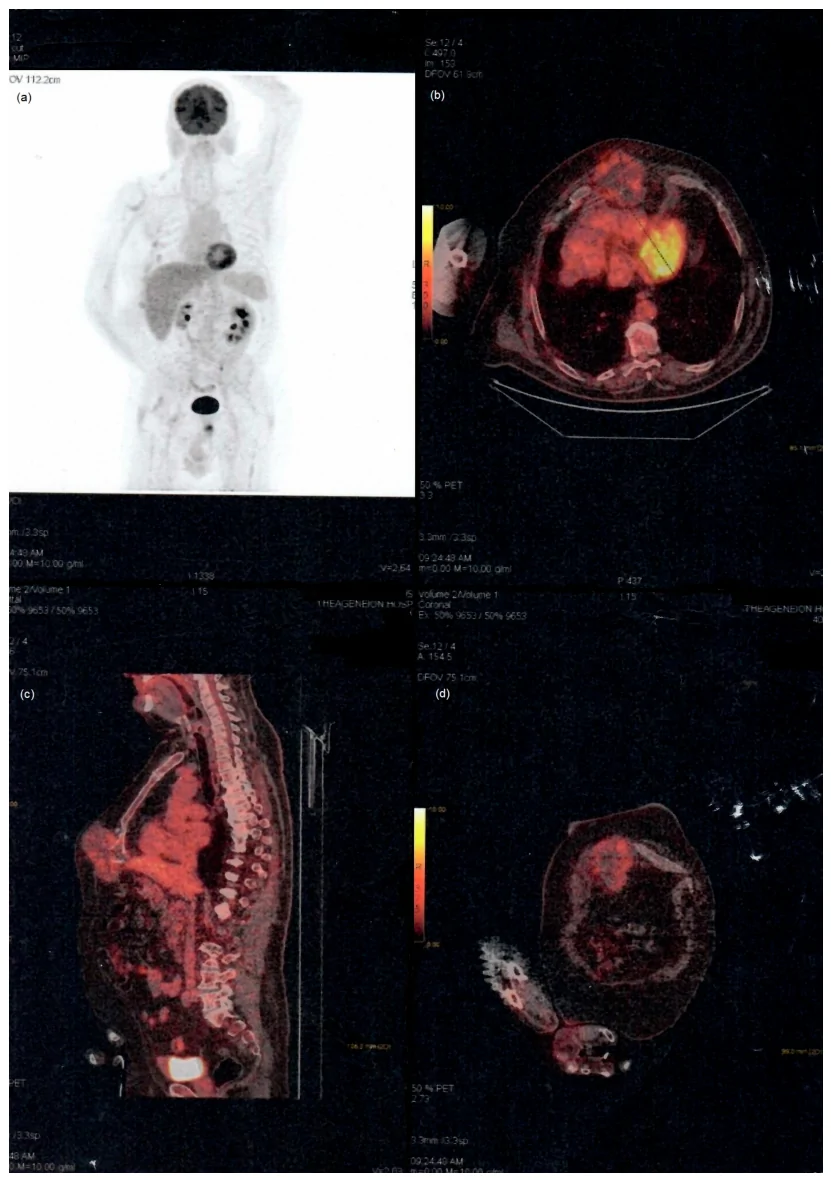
Multiplanar preoperative PET/CT imaging, including (a) Whole-body maximum-intensity projection PET caption in inverted grayscale, (b) Axial fused PET/CT caption through the lower thorax, (c) Sagittal fused PET/CT reconstruction and (d) Axial fused PET/CT caption at the level of the lower anterior chest wall/xiphoid region.
